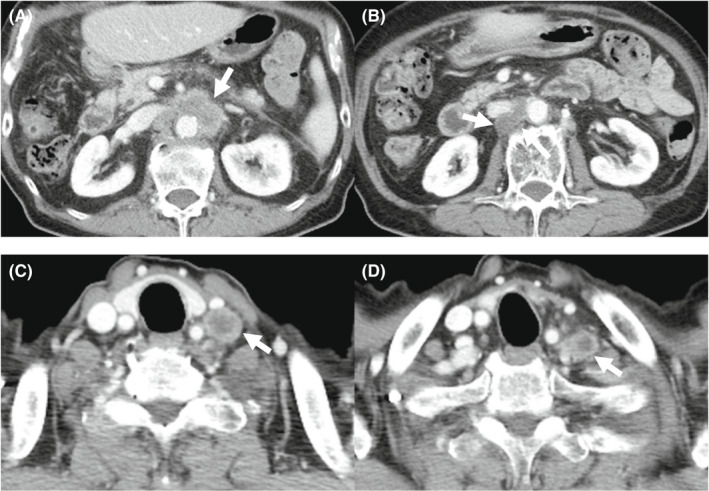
Enhanced computed tomography showed multiple lymph nodes metastases before Atez/Bev. The size of enlarged multiple lymph nodes remained almost the same and any new lesions were not detected. (A) Lymph nodes around the abdominal aorta. (B) A lymph node behind the inferior vena cava. (C, D) Left supraclavicular lymph nodes
